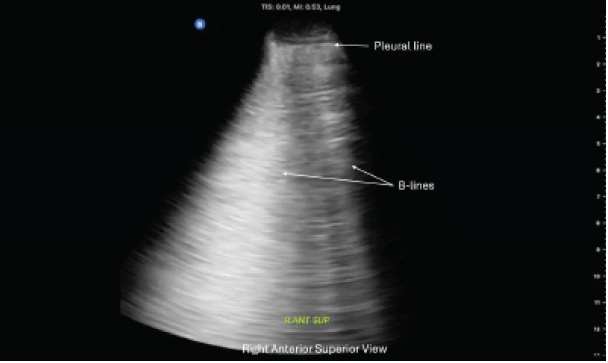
Point-of-care ultrasound of the right lung, in the right anterior superior view, showing numerous B-lines in a non-fatal drowning patient.
